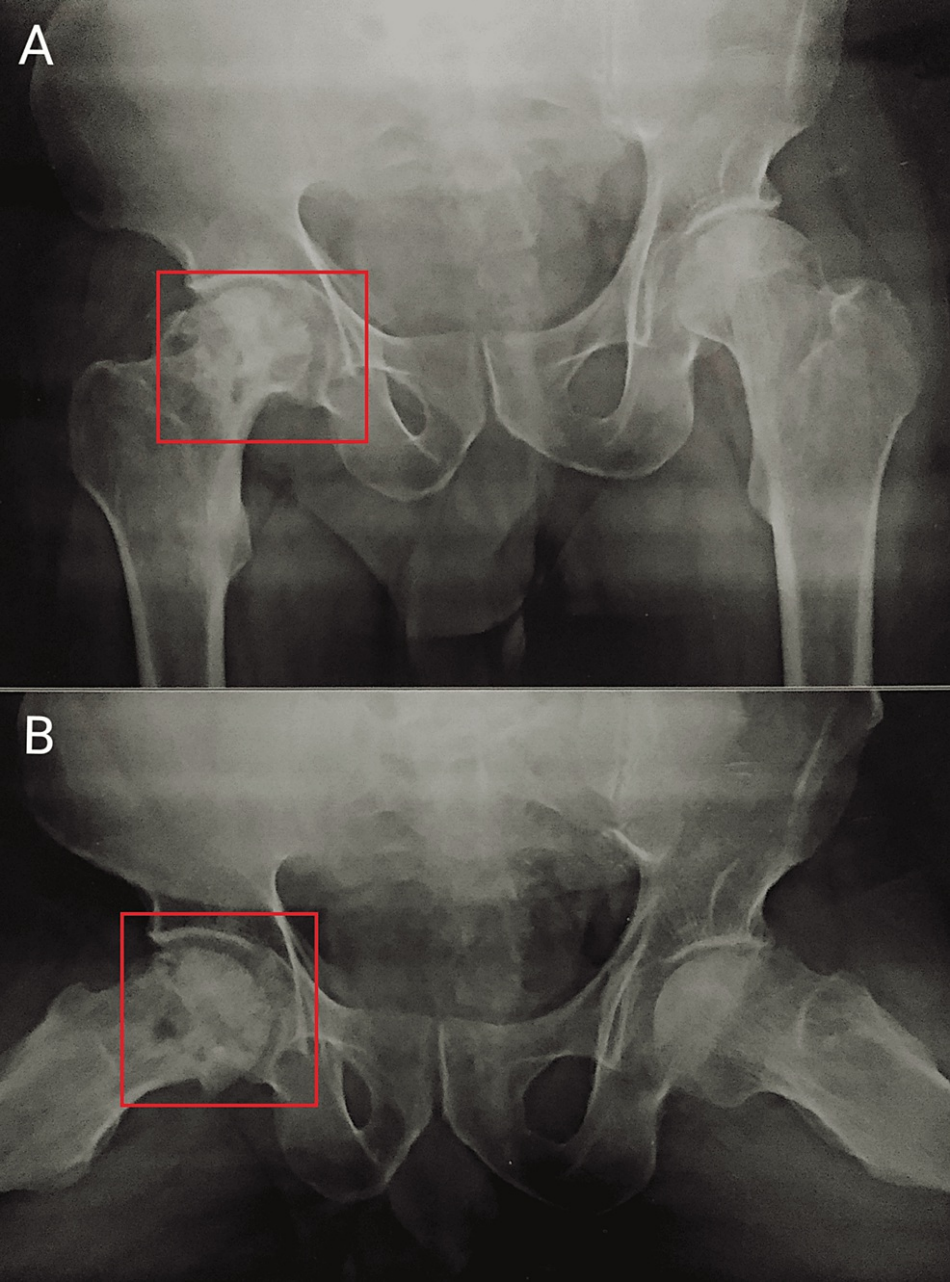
An X-ray from the anterior view of pelvis and hip joints. (A) and (B): Both the boxes on the right hip joint show reduced joint space.
